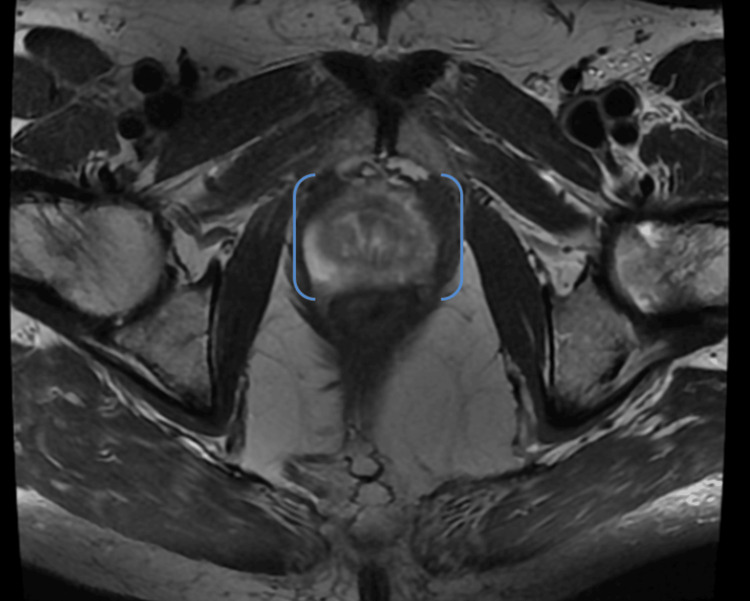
MRI pelvis showing prostate (bracketed in blue) with 15 mm PIRAD 4 focus noted in the left peripheral zone mid gland, with 82 mL gland volume and a PSA density of 0.062 PSA: prostate-specific antigen; MRI: magnetic resonance imaging; PIRAD: prostate imaging-reporting and data
